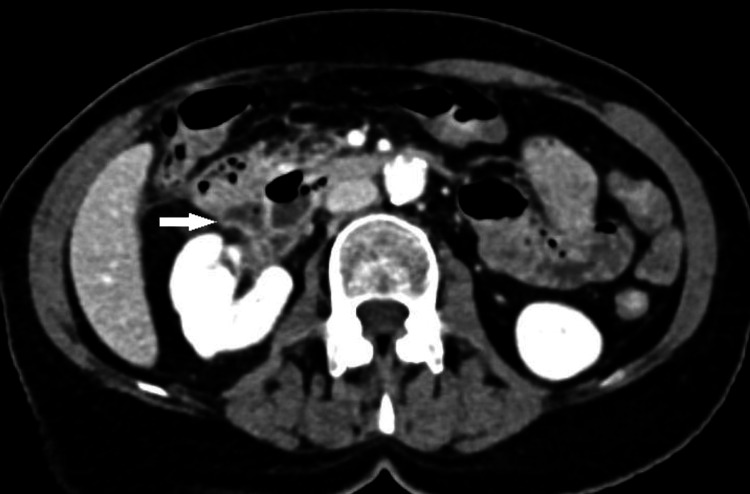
Follow-up CT of the abdomen and pelvis. Air-fluid collection near the posteromedial border of the descending duodenum with anterior pararenal fascia hypervascular thickening suggestive of incipient abscess formation (white arrow).
